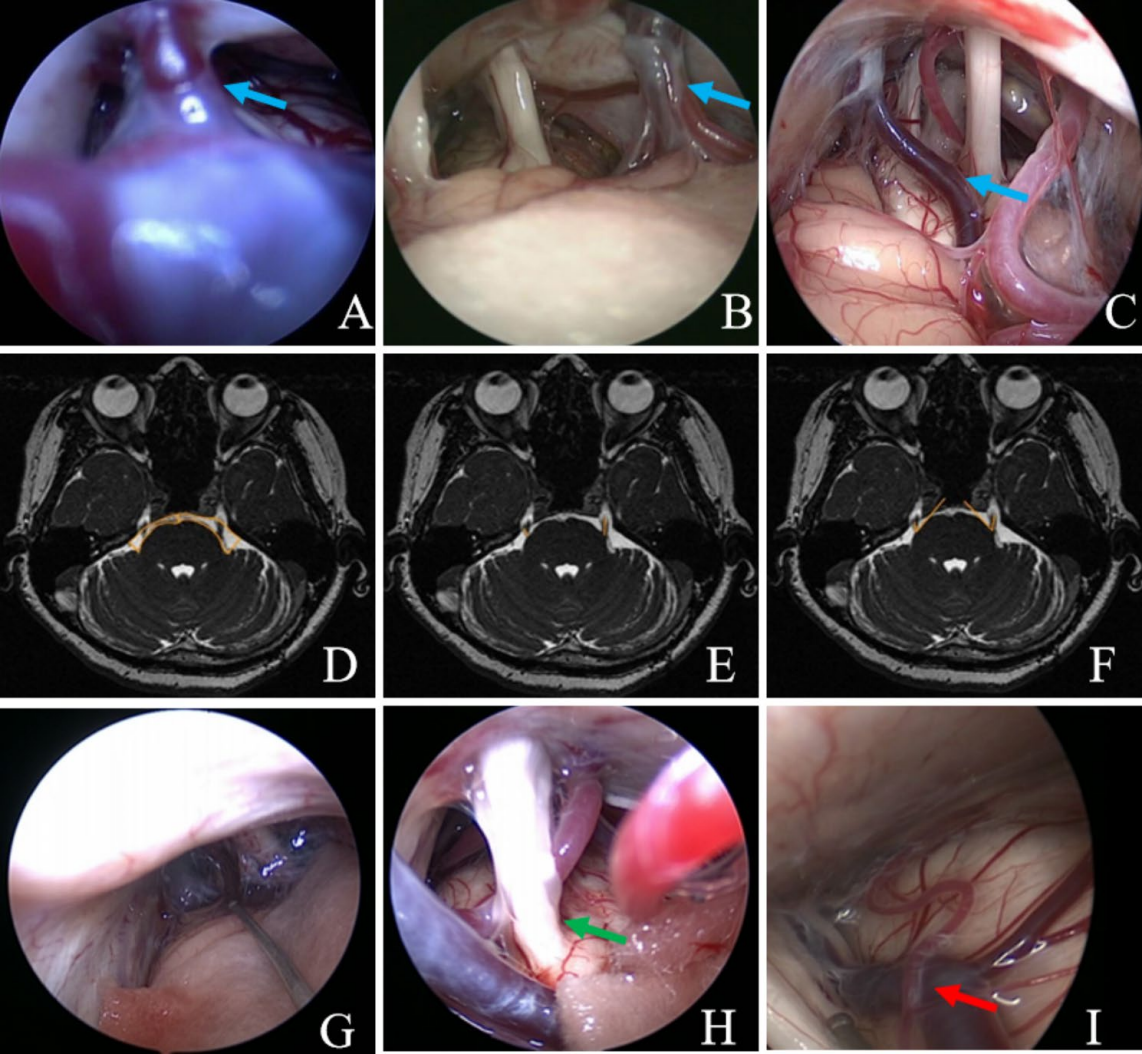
A-C, Clinical classification of PV (indicated by the blue arrow). (A) Type I: length < 5 mm and the tension is high; (B) Type II: length between 5 ~ 10 mm and the tension is moderate; (C) Type III : length > 10 mm and the tension is low. D-F, Preoperative 3D FIESTA MRI of patients with recurrent TN.(D) Area values of the CPA area bilaterally, the area of the CPA on the left side is larger than that on the right side, the area ratio of the CPA (healthy/afflicted side) = 1.67/0.91 = 1.84 > 1; (E) Length values of the TGN bilaterally, it can be seen that the length of the TGN on the left side is longer than that on the right side, the length ratio of the TGN (healthy/afflicted side) = 0.82/0.45 = 1.82 < 1; (F) TGN angle values, it can be seen that the angle of the left side is larger than that of the right side, the angle ratio of TGN (healthy/afflicted side) = 51.8/42.4 = 1.22 < 1. G-I, Intraoperative endoscopic images of the patient with recurrence. (G) The overall area of the CPA is narrower in the endoscopic field of view, which makes endoscopic manipulation difficult; (H) The responsible vessels are visible, forming obvious compression on the TGN, and the green arrow indicates severe deformation of the TGN due to long-term vascular compression; (I) The red arrow indicates simultaneous arterial and venous compression of the TGN
